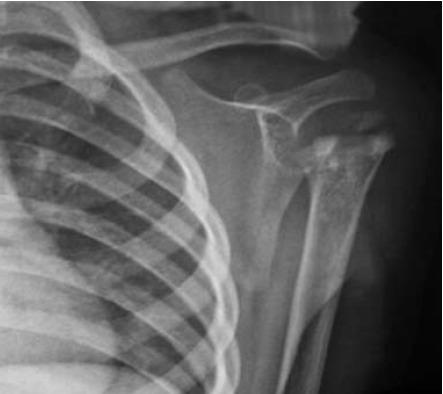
Fig. 3: Radiograph of left shoulder at 6 weeks demonstrates marked improvement of the proximal humerus of the left shoulder in comparison with radiographs taken at 2 weeks. F3:
